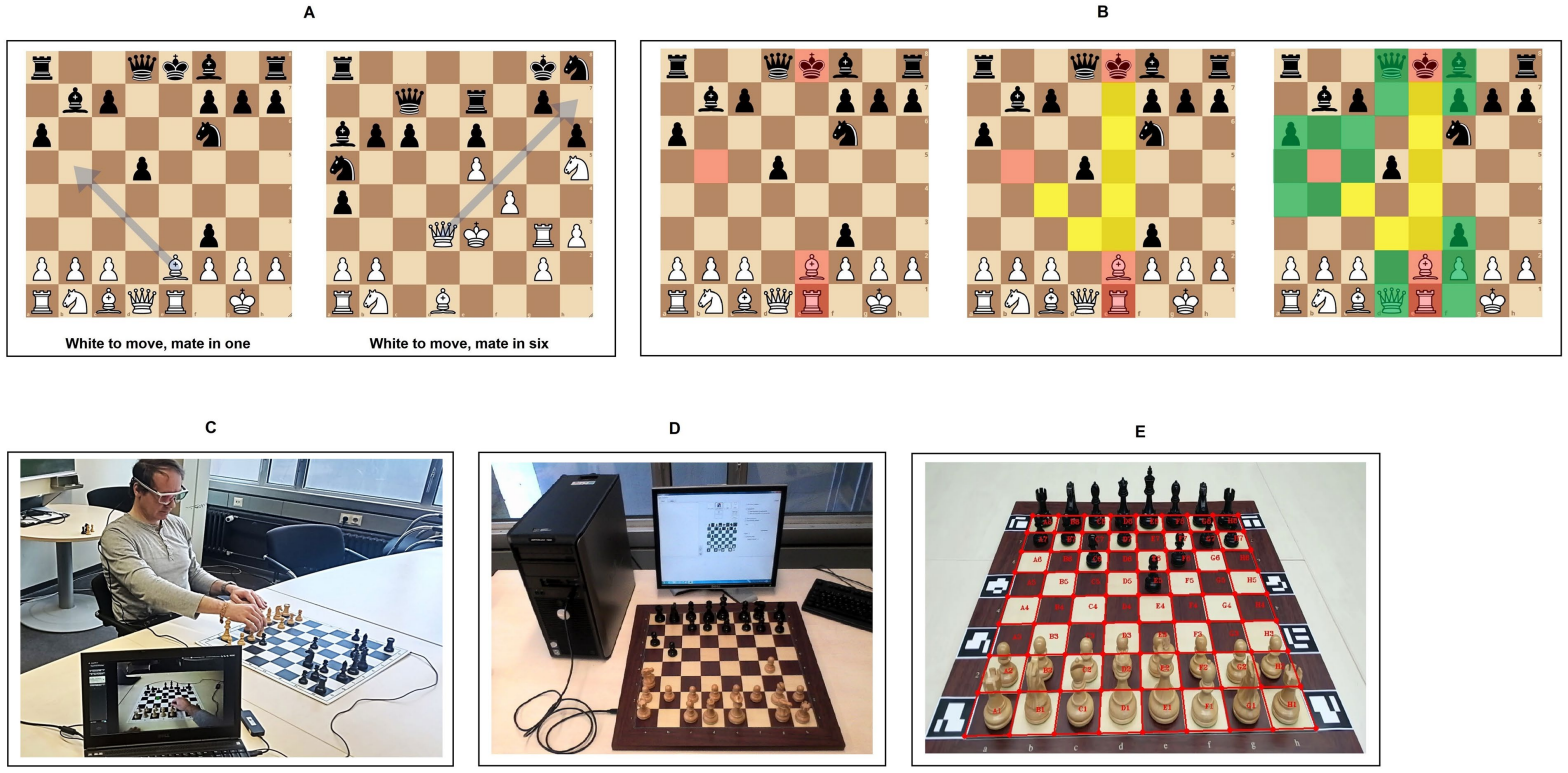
(A) Examples for n-mate tasks: Task 1 “White to move, mate in one” and Task 11 “White to move, mate in six”; (B) Three different types of AOIs—explained on the basis of Task 1. Left side: AOI 1 (red) includes the attacked king, both attackers bishop on e2 and rook on e1 and the target square b5 of the bishop’s attacking move; middle: AOI 2 includes AOI 1 plus the bishop’s piece replacement squares (yellow) and squares X-rayed (yellow) by the rook; right side: AOI 3 includes AOI 1 and AOI 2 plus the nearest neighbour squares (green) of all AOI 1 squares; (C) Experimental setting demonstration: Chess player whose gaze is recorded by SMI eye tracking while solving Task 1; (D) The DGT e-board connected to a screen and a computer via USB; (E) Implementation of eight ArUco markers to stabilise the chessboard detection.
